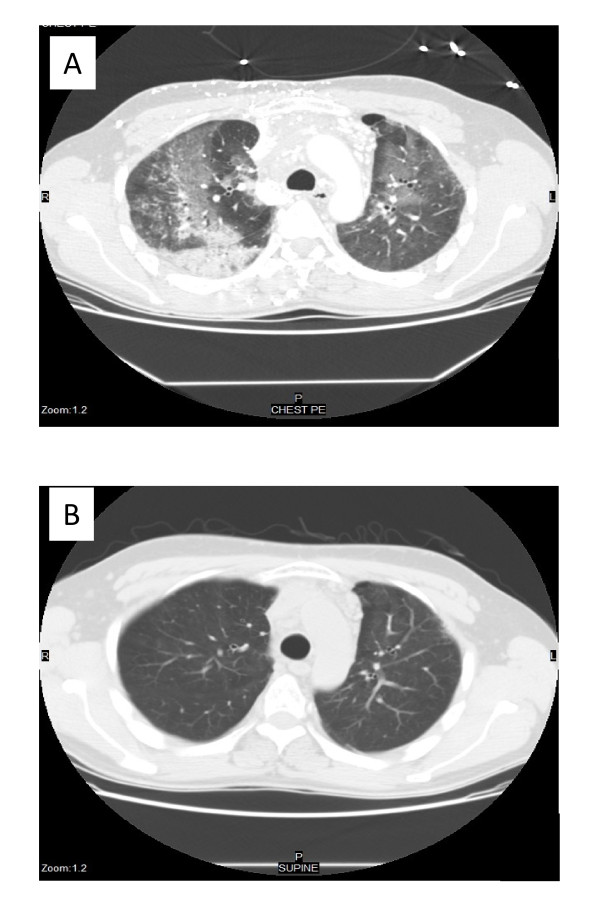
Pulmonary infiltrates before and after steroid treatment. (A) Computed tomography (CT) of chest with intravenous contrast in March 2007 showing right upper lobe, right middle lobe and left lung with patchy ground-glass opacities. (B) CT of chest one month after steroid treatment showing complete resolution of ground-glass opacities in both lung fields.
